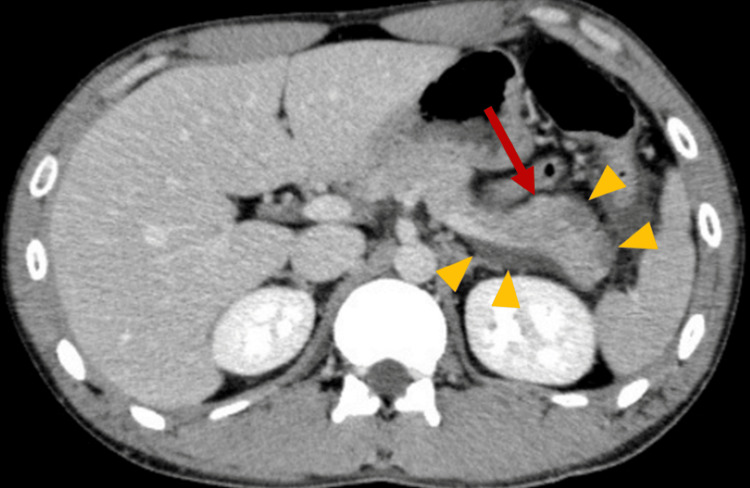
Contrast-enhanced CT showing a poorly enhanced area in the pancreatic tail, suggestive of inflammation and ischemia (red arrow), and inflammatory changes around the pancreas (yellow triangles).
